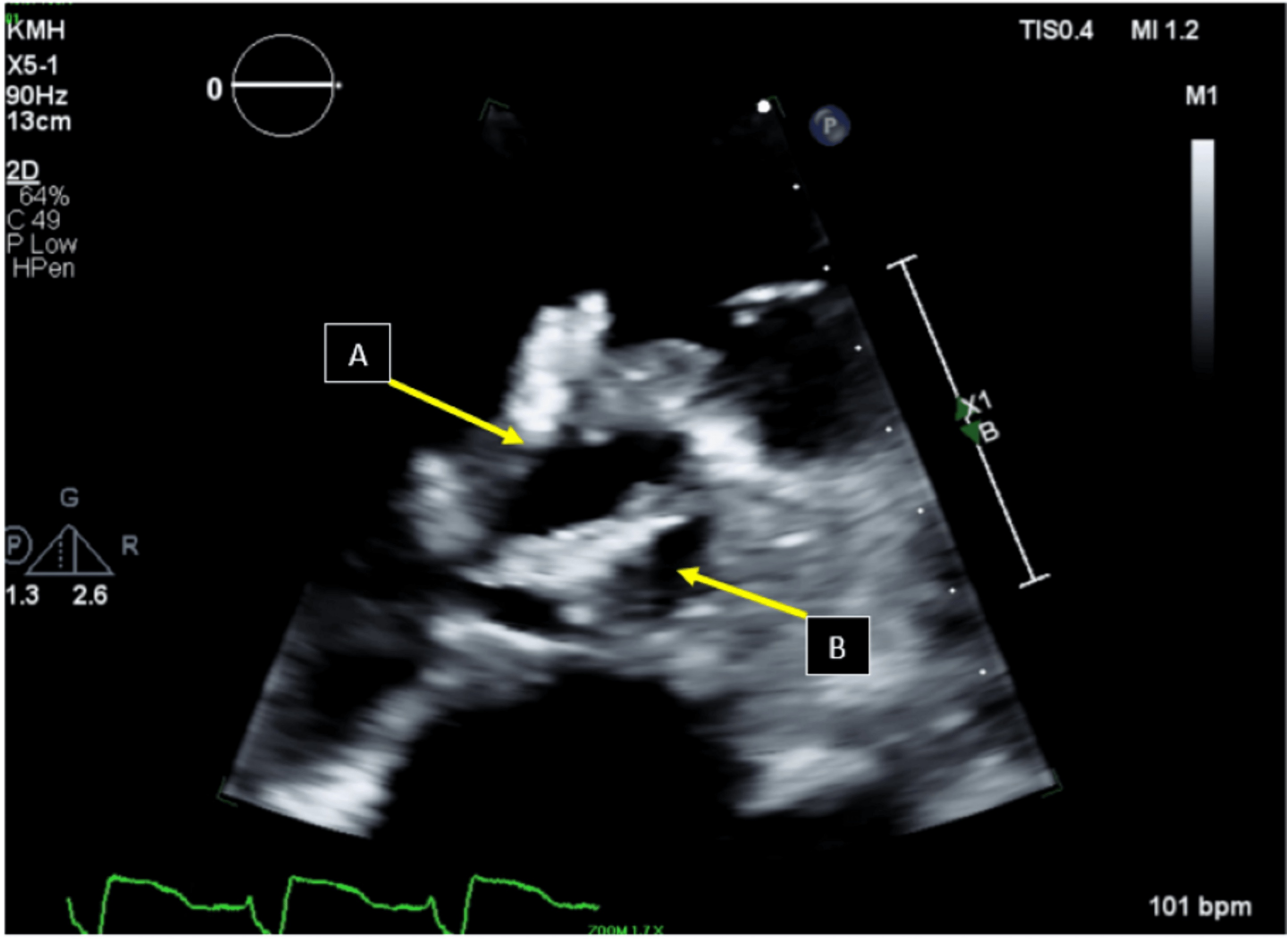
Transthoracic echocardiography (TTE) findings TTE showing a short-axis view of the aortic valve, showing large vegetations on the right coronary cusp (arrow A: RCC). Arrow B shows a large vegetation on the non-coronary cusp (arrow B: NCC).
